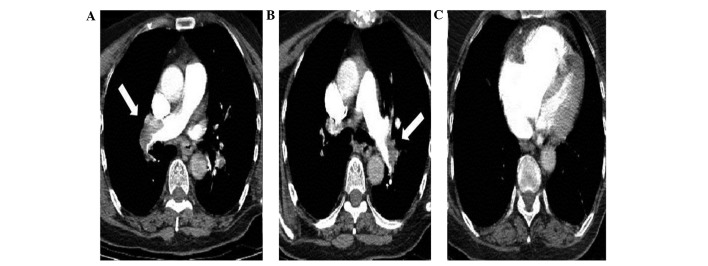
Pulmonary thromboembolism on computed tomography scan. (A) Right middle and lower pulmonary artery branch emboli. (B) Left pulmonary artery emboli. (C) Right ventricular dilatation (right ventricular cavity wider than left ventricular cavity in short axis).
